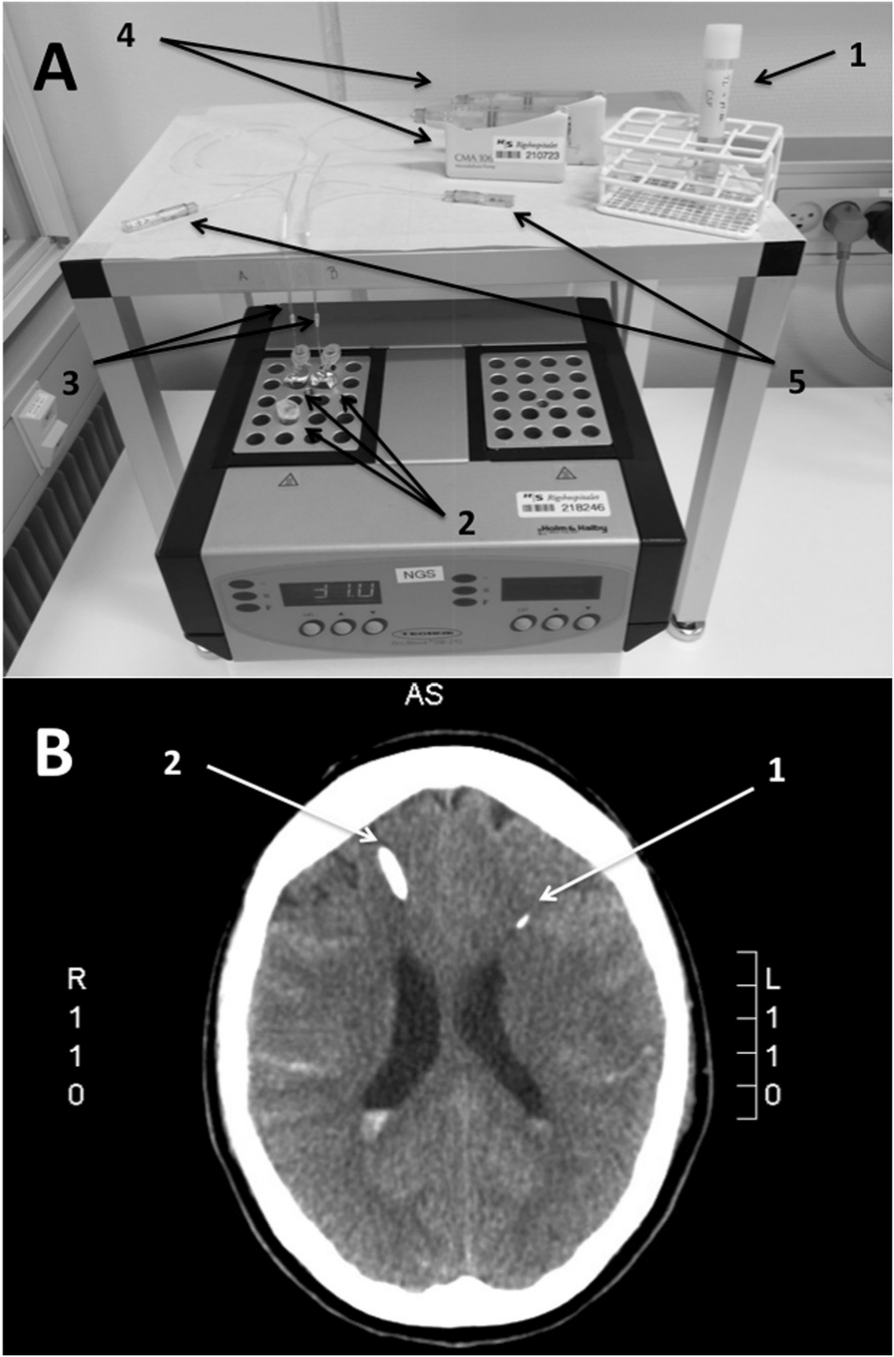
Materials and methods. A: Setup for *in vitro* microdialysis. 1, cerebrospinal fluid sample; 2, identical aliquots of the cerebrospinal fluid; 3, two microdialysis catheters with a membrane length of 10 mm and a 20 kDa cut-off; 4, perfusion pumps; 5, vials gathering samples of microdialysis fluid. B: Computed tomography scan from SAH patient 3. 1, a microdialysis catheter placed in the left frontal lobe; 2, part of the external drain passing through the brain.
